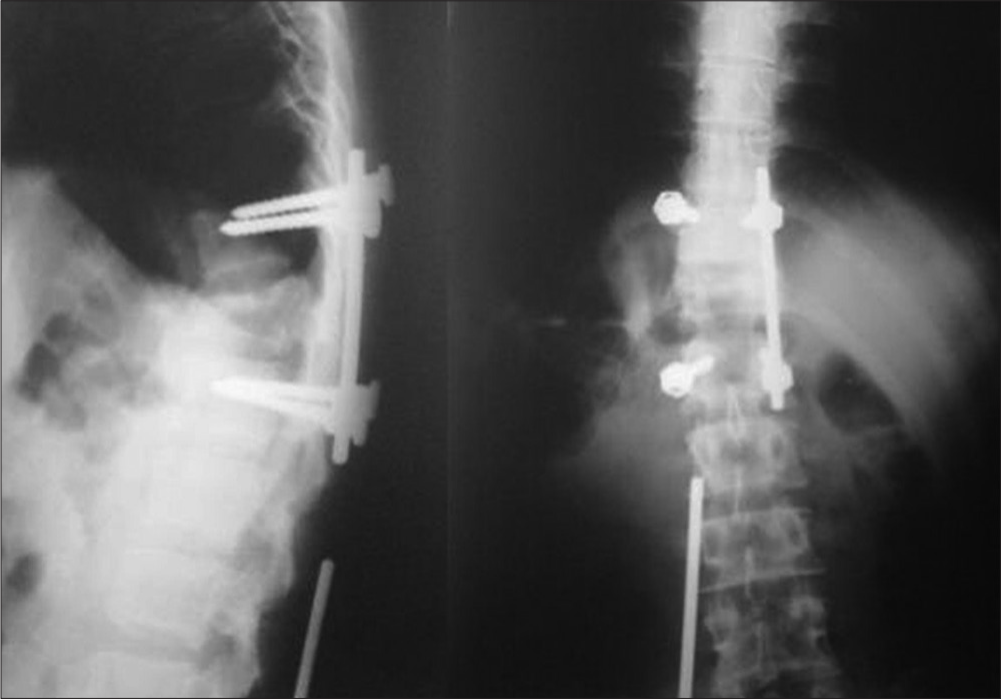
Radiograph revealing loosening of implant and migration of connecting rod
